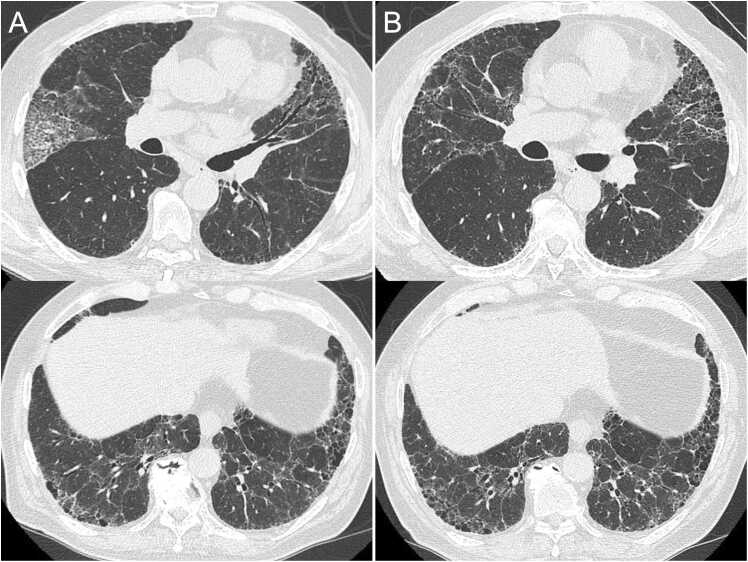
High-resolution computed tomography (HRCT) images. (A) Bilateral peripheral ground-glass opacities with a characteristic three-density pattern, obtained during relapse while tapering corticosteroid therapy. (B) One year after antigen avoidance and antimycobacterial therapy, the patient showed improvement in the middle lobe opacity.
